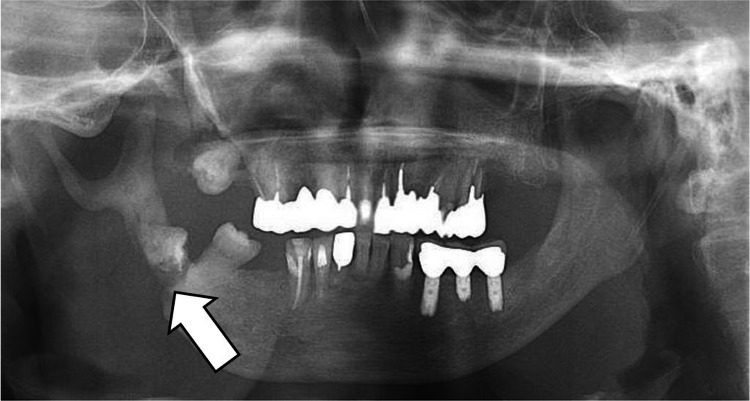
Panoramic radiograph of the patient Pathological fractures associated with mandibular osteomyelitis
